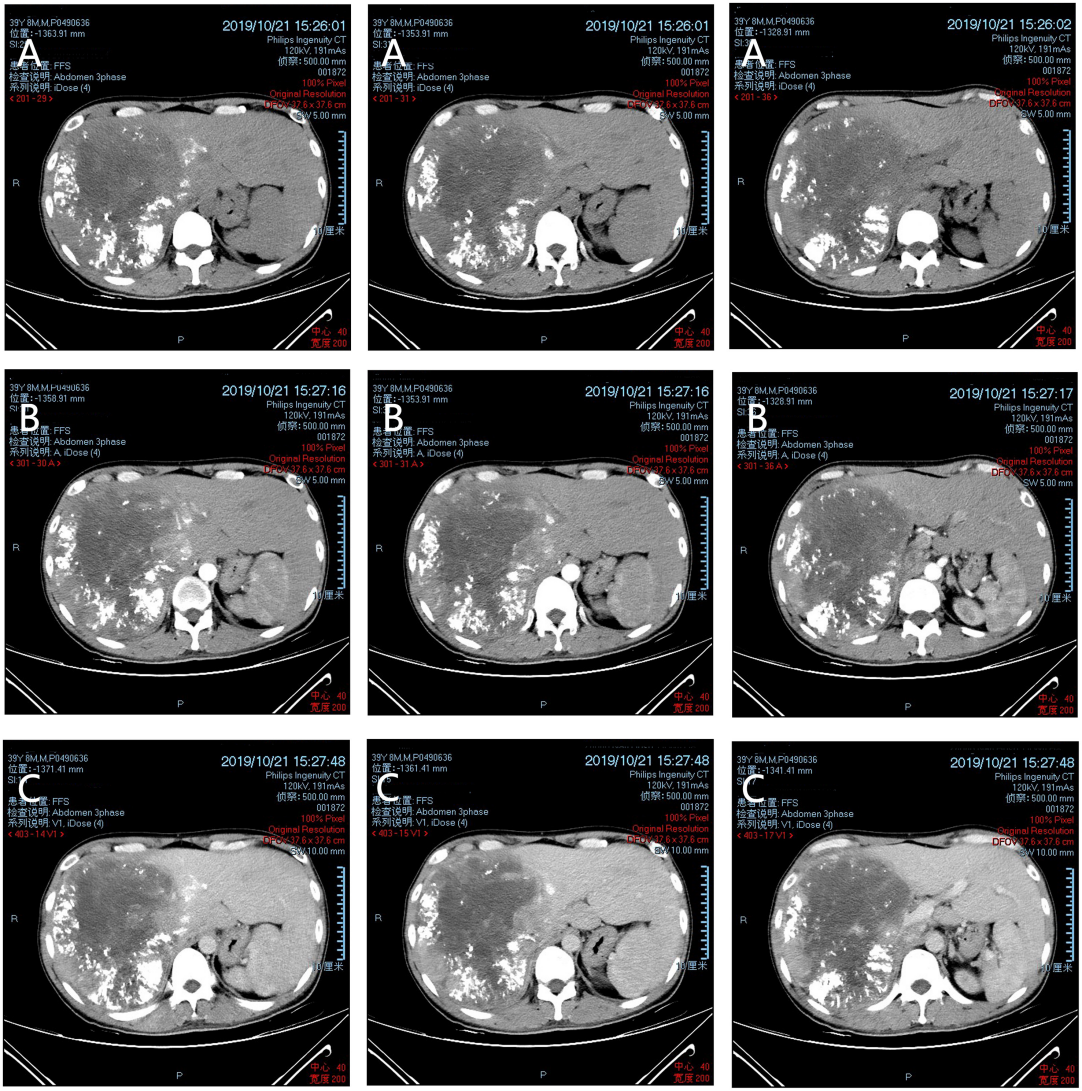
CT images after TACE. (A) Plain scan; (B) arterial phase; and (C) portal venous phase.
